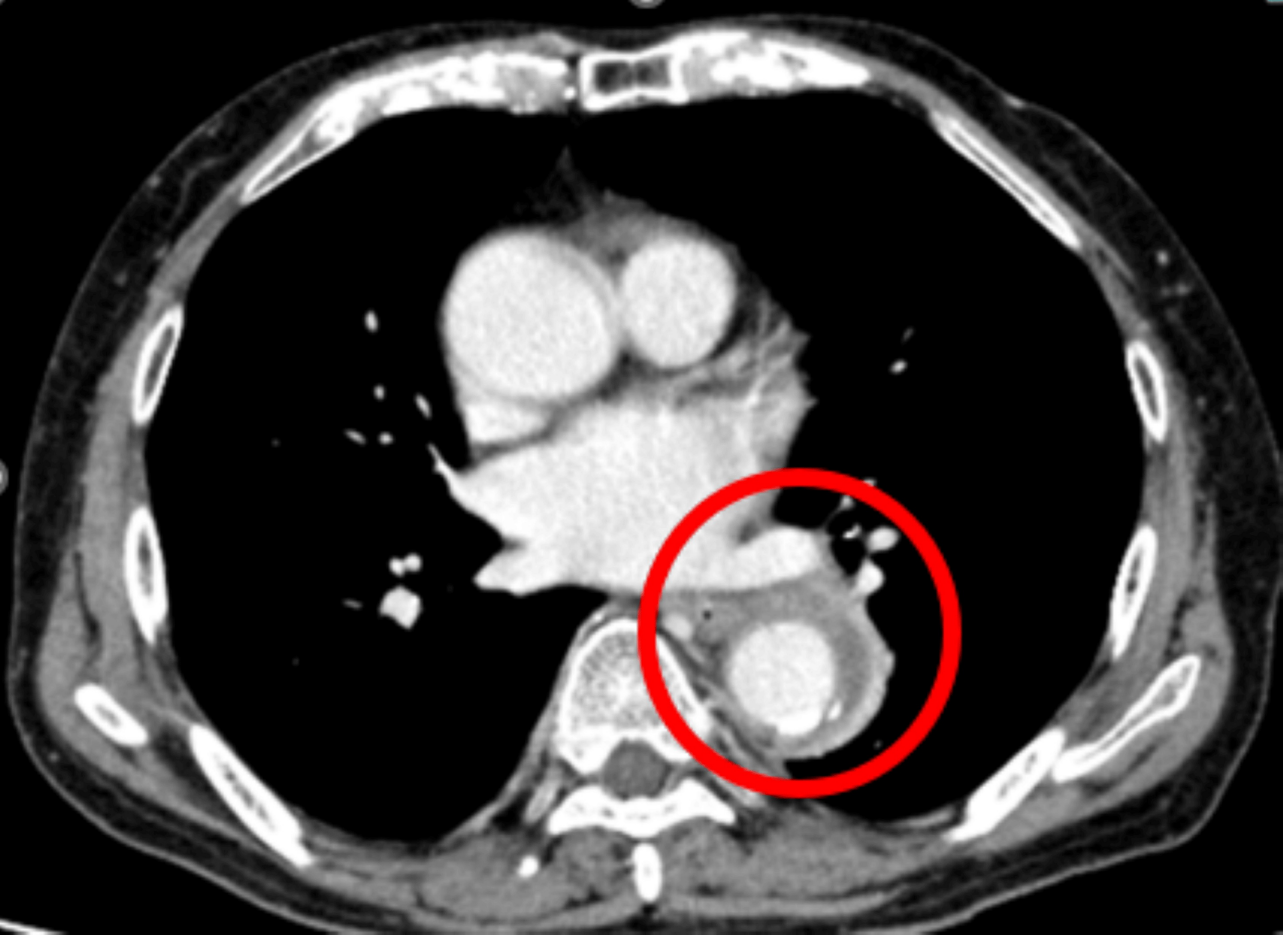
The horizontal slice of chest contrast enhanced CT on the day of admission The soft tissue density is seen around the descending thoracic aorta with mildly elevated adipose tissue density around the aorta (red round).
